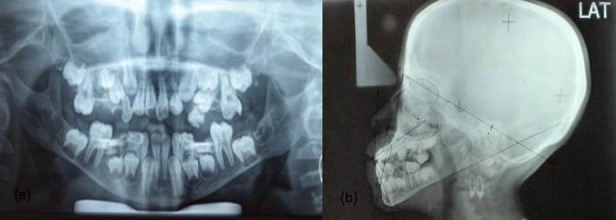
Extraoral radiographs: a: Orthopantomogram, b: Cephalometric tracing (Steiner’s analysis)
